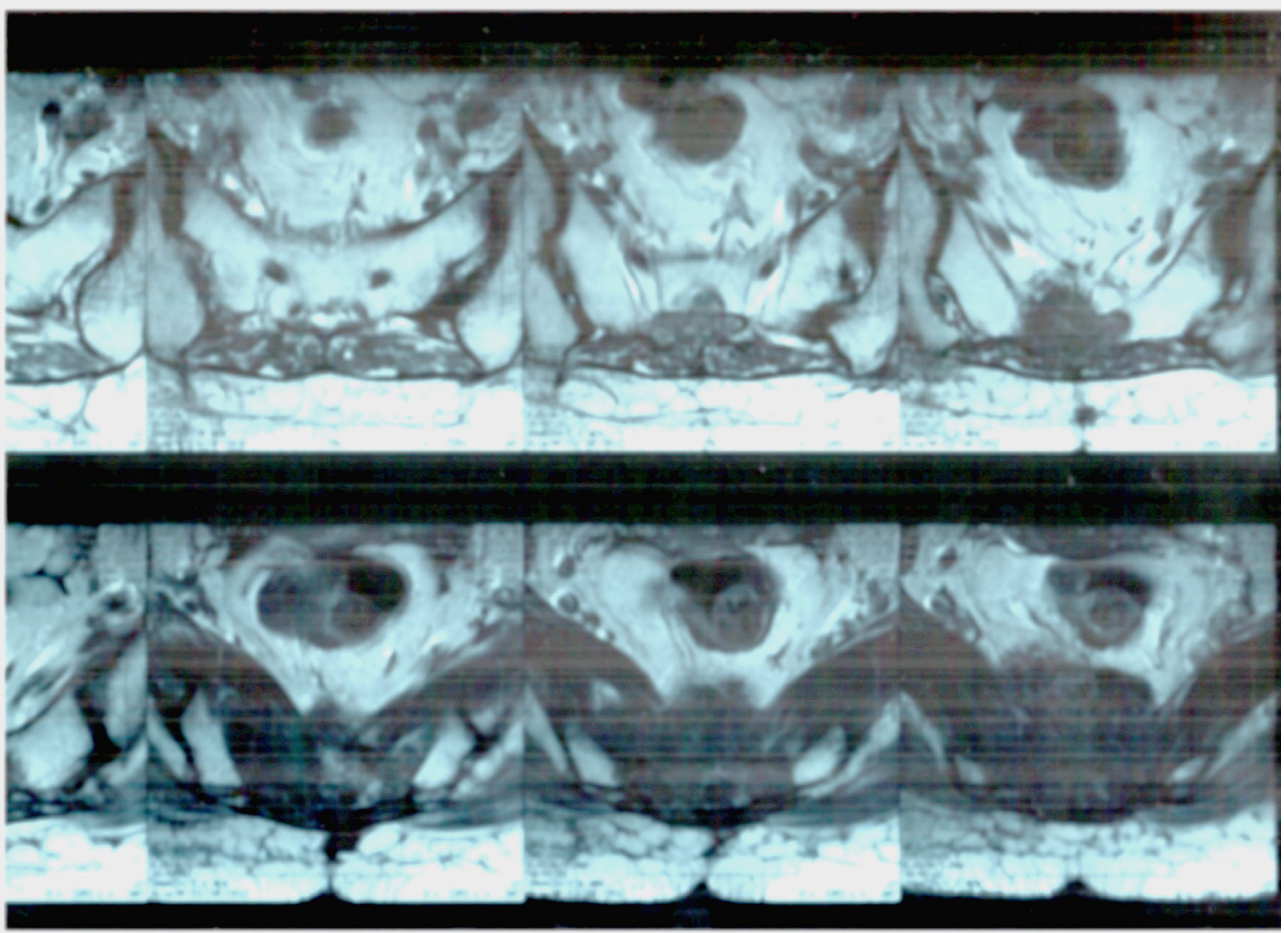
Pelvic CT scan in the patient with chordoma shows the lesion infiltrating the sacrum.
